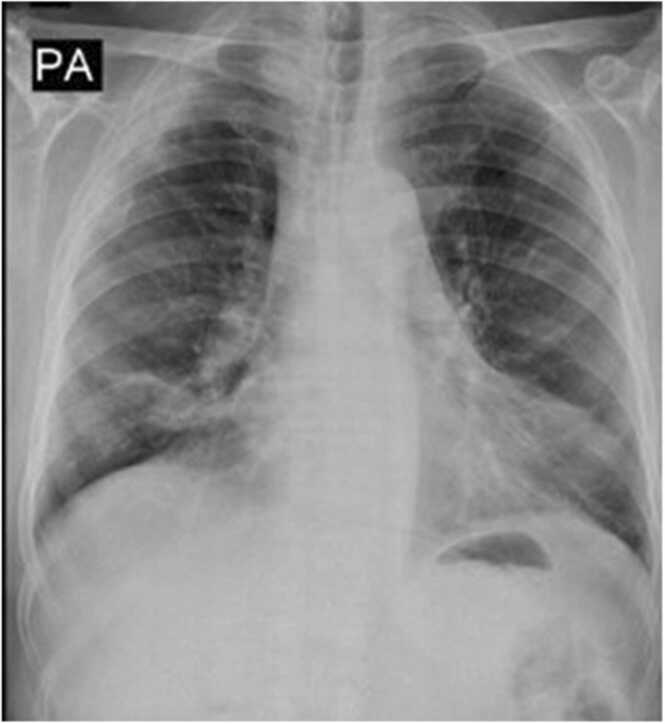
Chest X-ray posterior-anterior view showing bilateral nonhomogeneous opacities in the lower zone.
